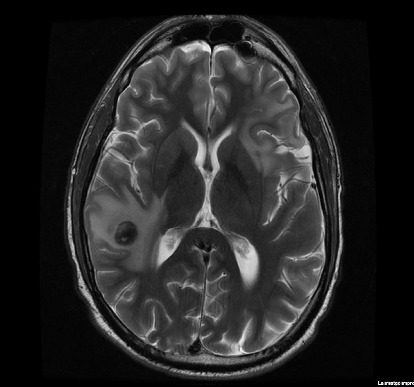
Brain MRI response. Axial view revealed the presence of intra-axial lesions, one at the right temporal region (with evident perilesional edema 15 mm in diameter) and another at the left frontal region in the cortico-subcortical area with marked perilesional edema. Lesions showed low signal intensity on T2-weighted images and FLAIR.
